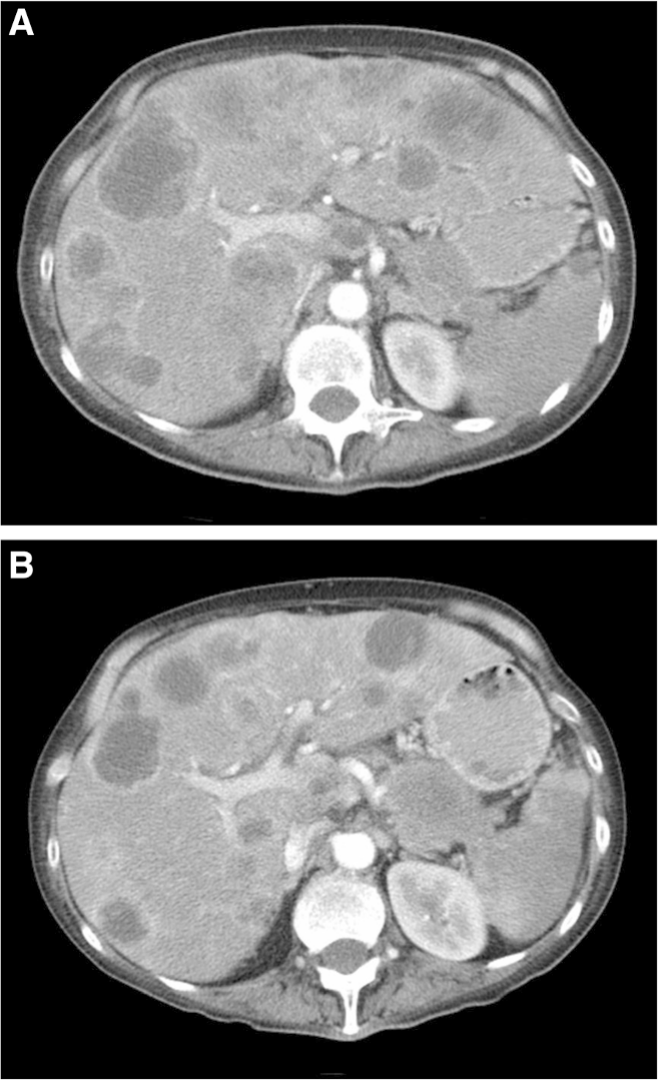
Tumour response in a patient with liver-only metastases from primary pancreatic adenocarcinoma. a Contrast-enhanced CT scan prior to SIRT + 5FU. b) Follow-up contrast-enhanced CT scan 3 months post-SIRT + 5FU, and prior to gemcitabine, demonstrates a partial response (40 % reduction in hepatic tumour burden), as assessed according to RECIST v1.0
